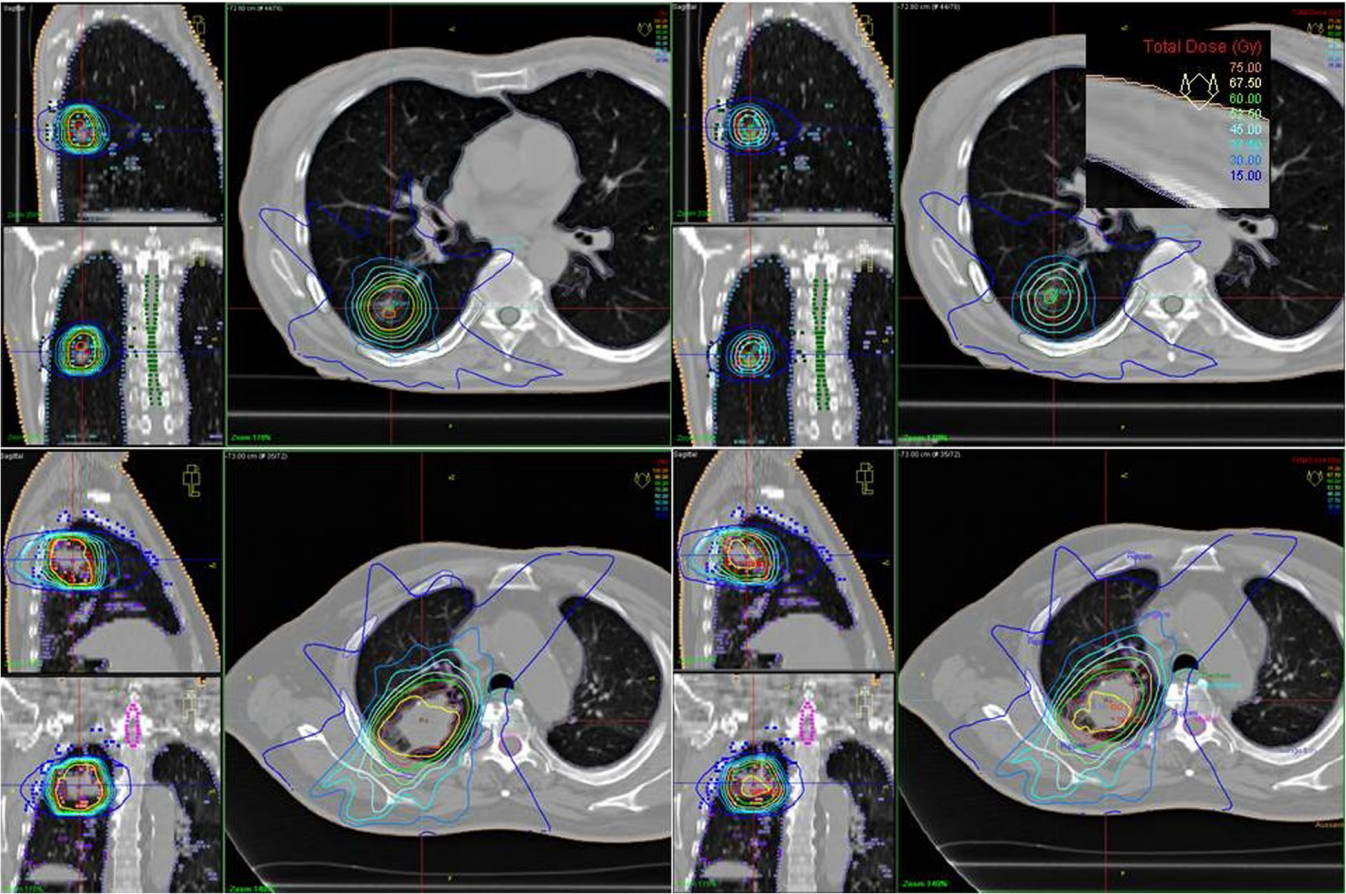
Sagittal, coronal and transversal CT view of two sample patients. The top slices show the most extreme case (Patient 2) due to the small tumor volume, whereas the bottom slices depict a less extreme case. Pencil beam (PB) is shown on the left and the plans recalculated with collapsed cone (CC_re-calc_) on the right. The orange, yellow, bright green, light green, light blue, medium blue, turquoise and dark blue lines represent the 75 Gy, 67.5 Gy, 60 Gy, 52.5 Gy, 45 Gy, 37.5 Gy, 30 Gy and 15 Gy isodoses, respectively
